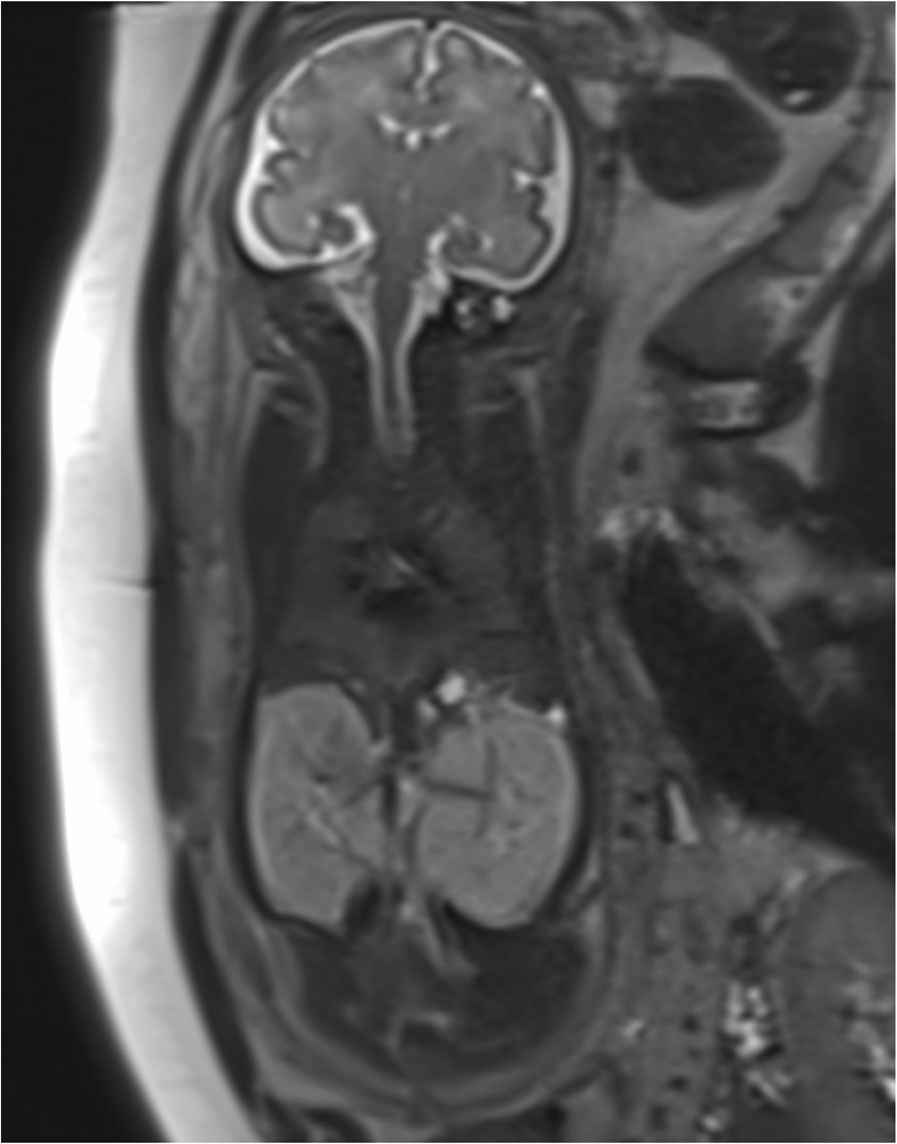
Fetal magnetic resonance imaging at 31 weeks of gestation. Coronal T2 image shows abdominal cavity occupied by two large hyperechogenic kidneys (5.5 cm) and decreased thorax size
